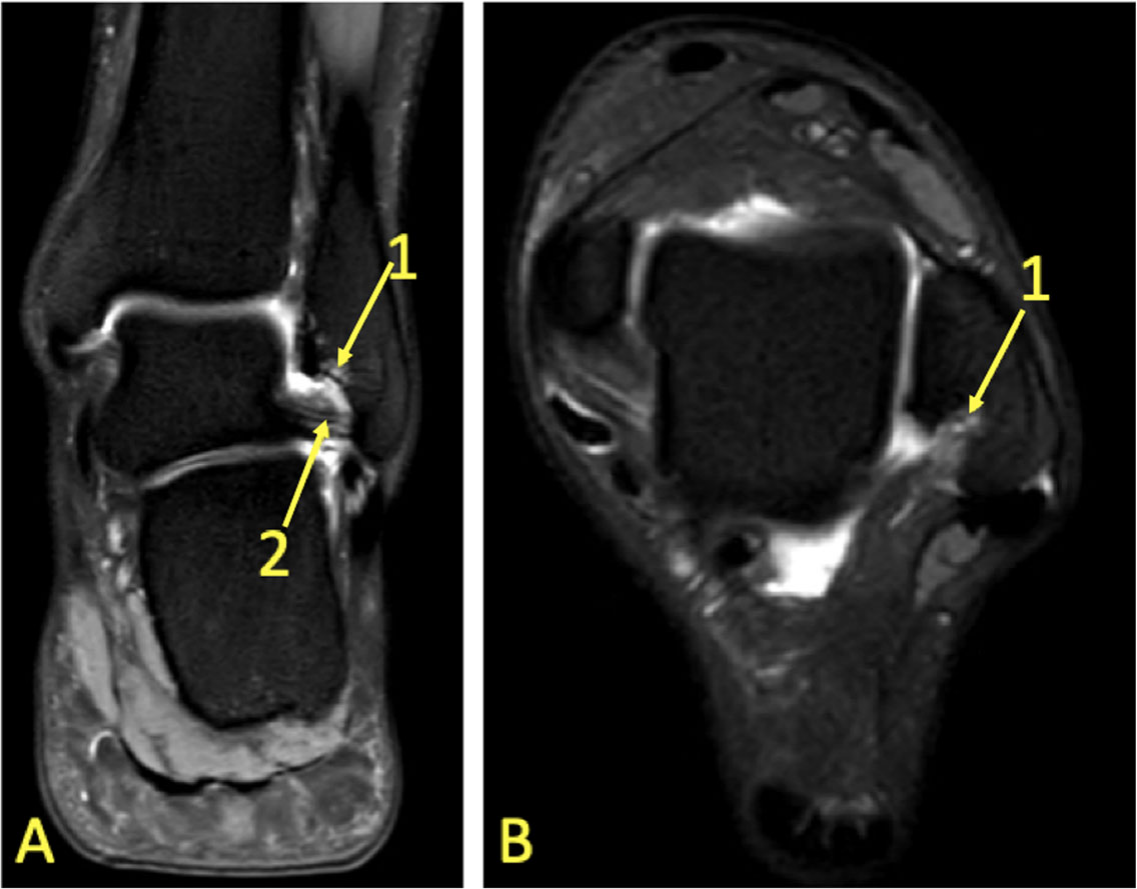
A 42-year-old male with 9-month history of ankle pain and suspicion of an osteochondral lesion. MRI (a, b) revealed traction cysts at the insertion of the posterior talofibular ligament
